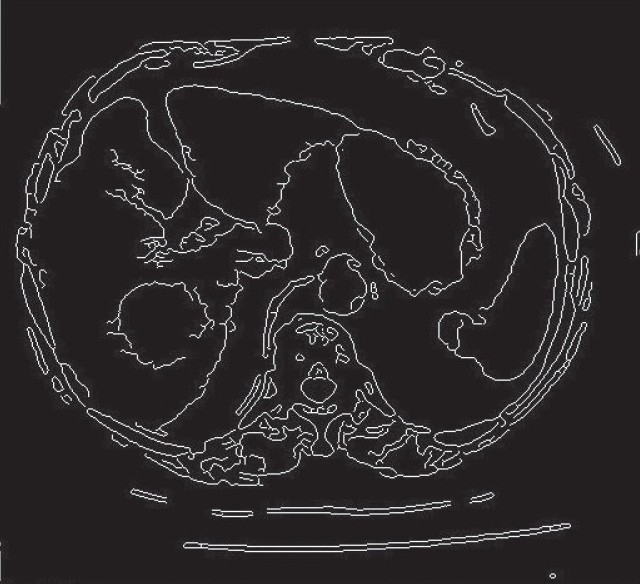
Result of Edge-based Segmentation of Abdomen (CT image)
